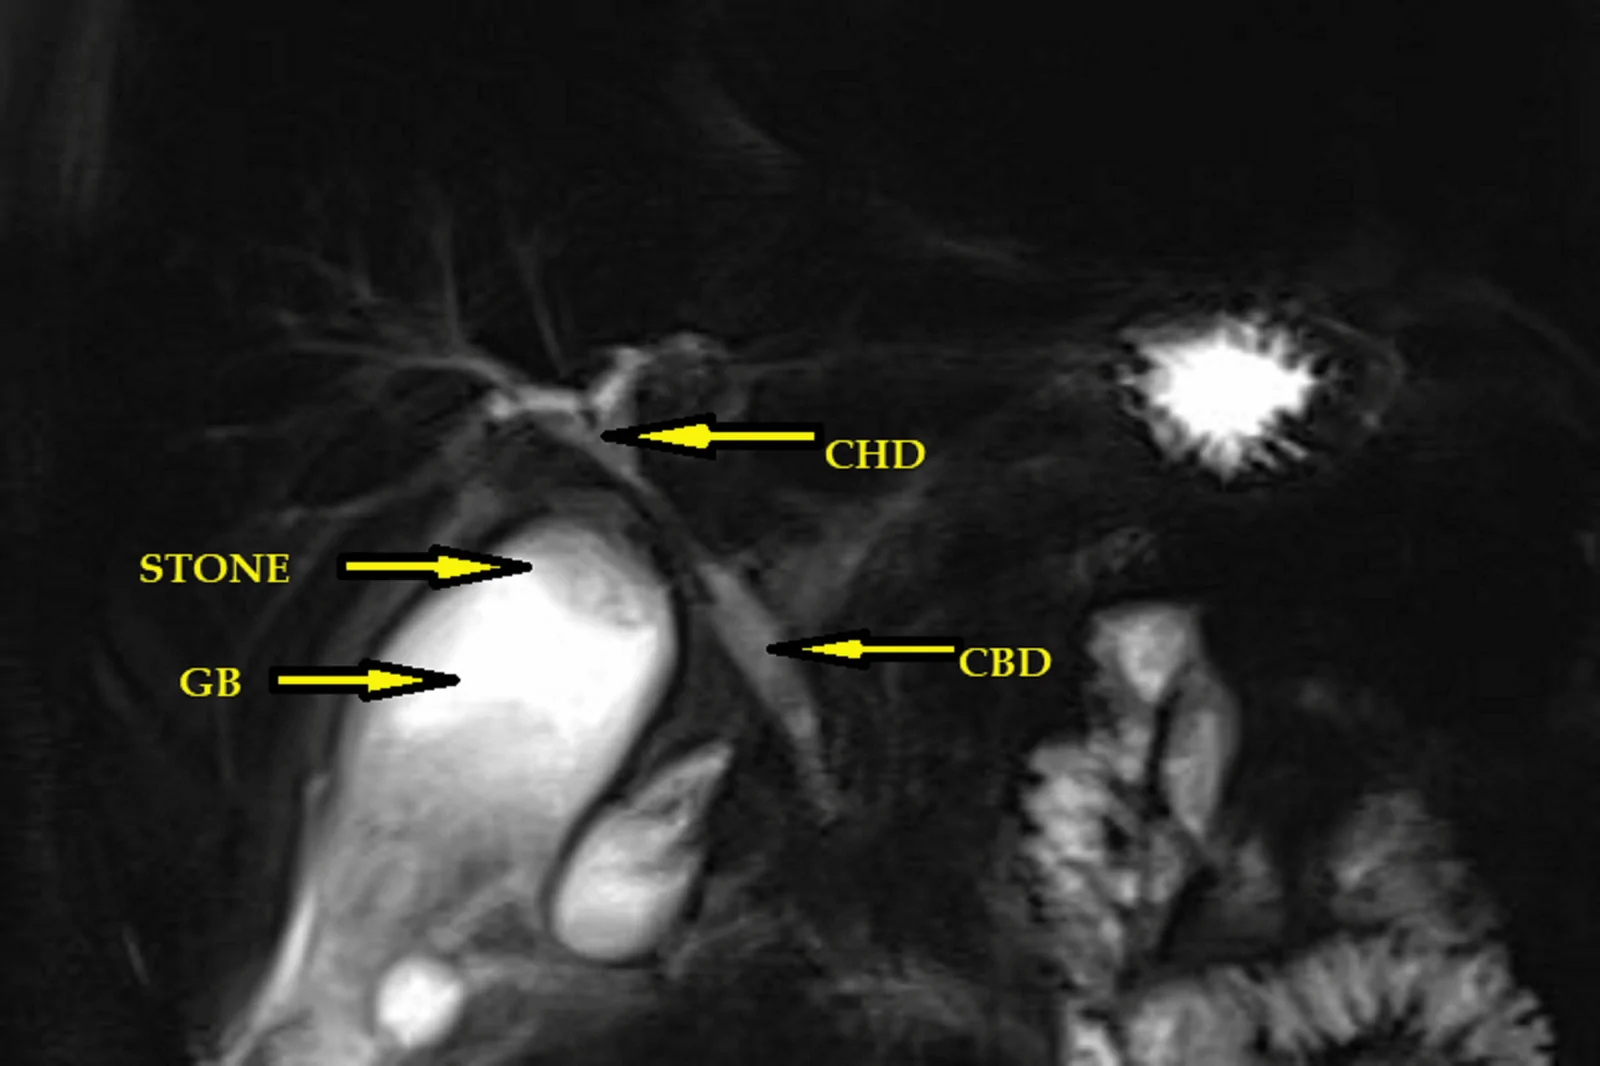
MRI showing the gallbladder (GB), stone, common hepatic duct (CHD), and common bile duct (CBD).
